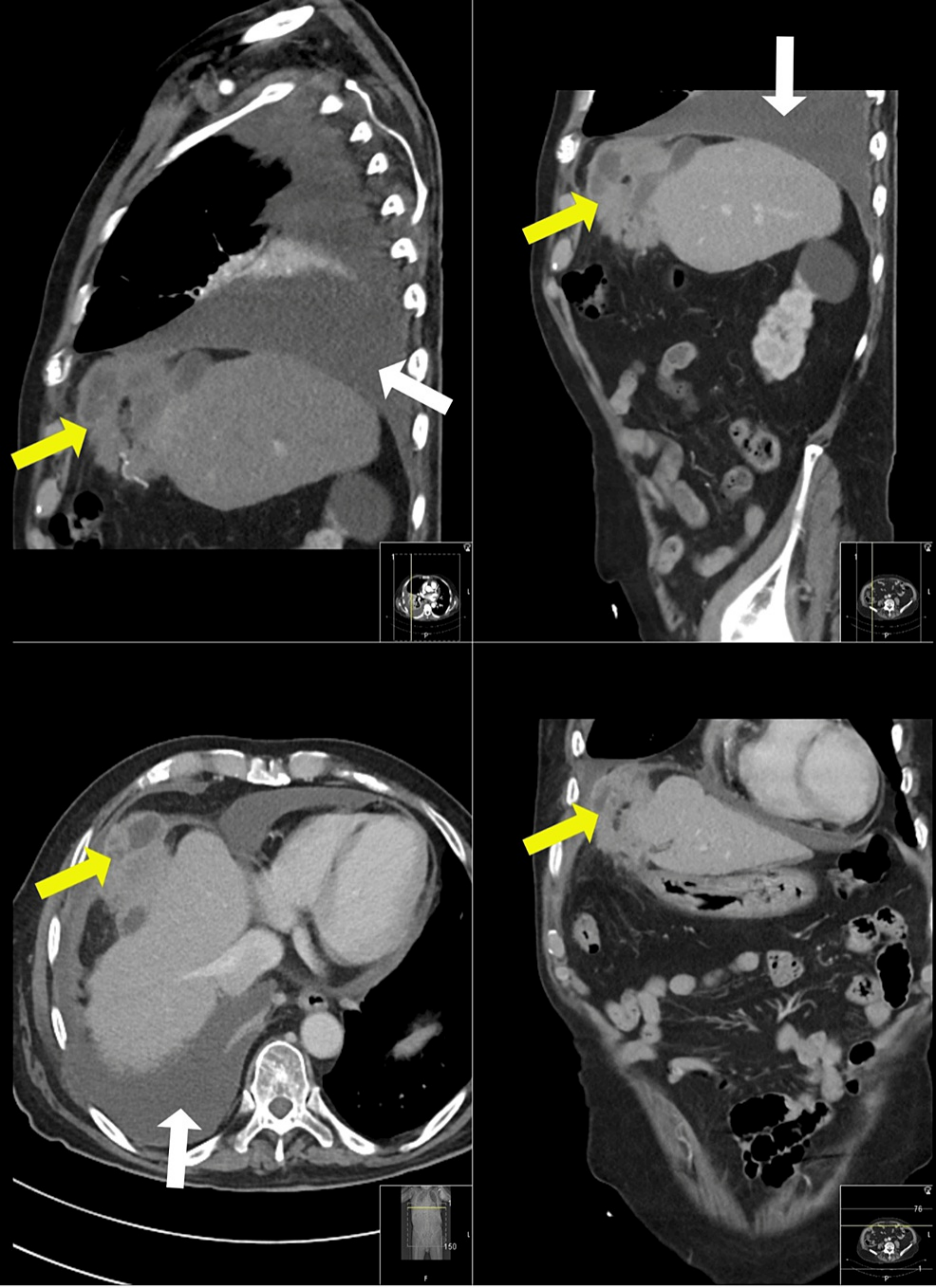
CT shows pericardial/pleural effusions, right lower lobe collapse (white arrows), and abnormal density at the right hemidiaphragm (yellow arrows).
